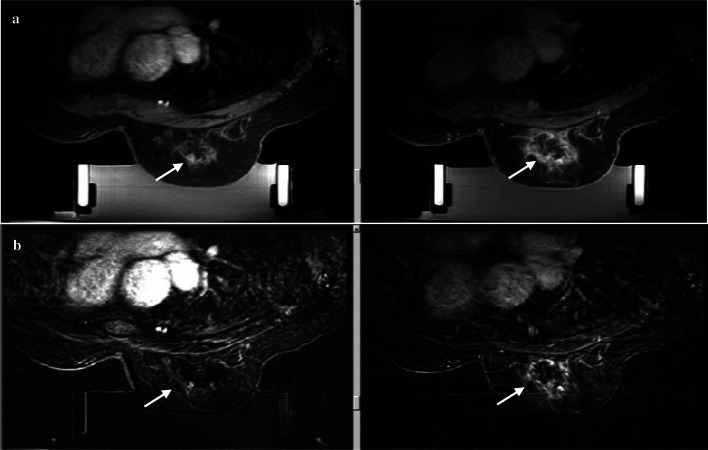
Enhanced magnetic resonance imaging (MRI) before and after MRgFUS treatment. Low-intensity area after ablation indicated tissue necrosis. High-intensity area indicated edema change of the tissue. White arrows indicate the change of the ablated area before and after MRgFUS treatment. a T1-weighted imaging of a 70-year-old-woman before (left) and after (right) ablation. b T1-weighted imaging of a 69-year-old-woman before (left) and after (right) ablation
